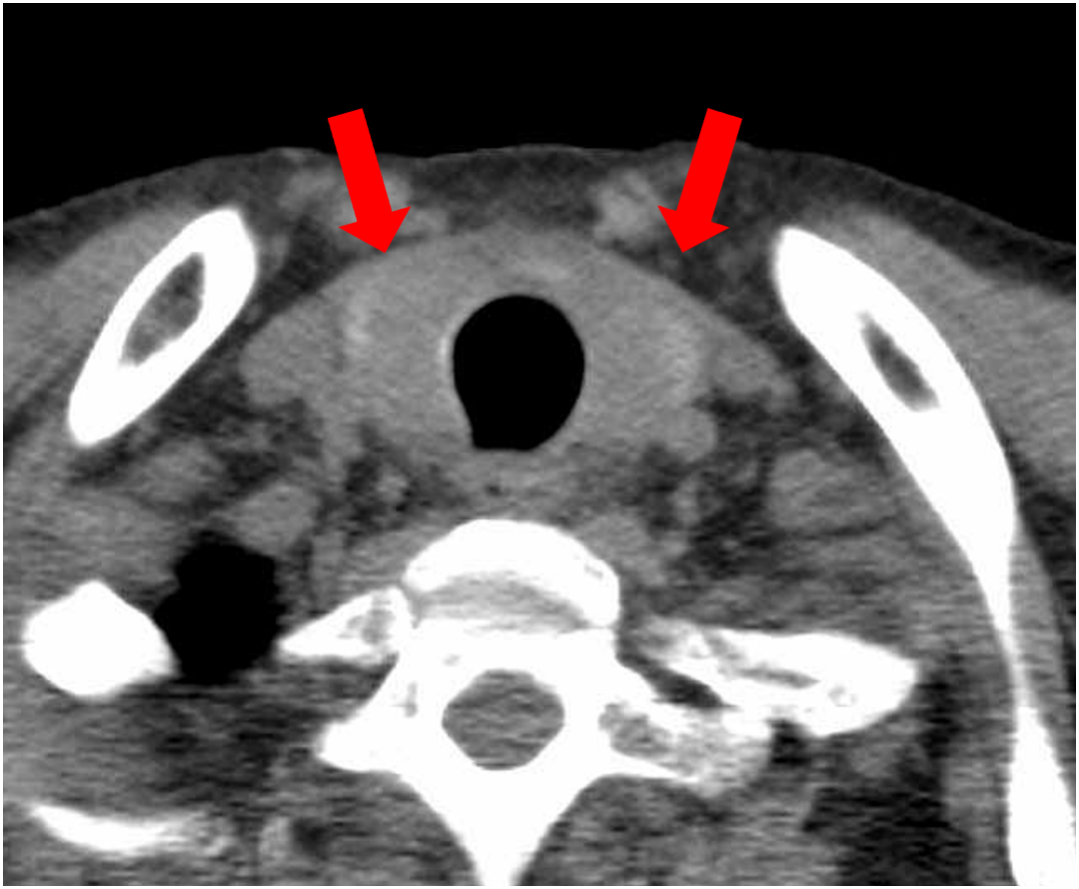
Computed tomography imaging of the thyroid on day 12 of admission. Low attenuation of the thyroid gland (red arrows) and enlarged thyroid indicated abnormal thyroid function.
